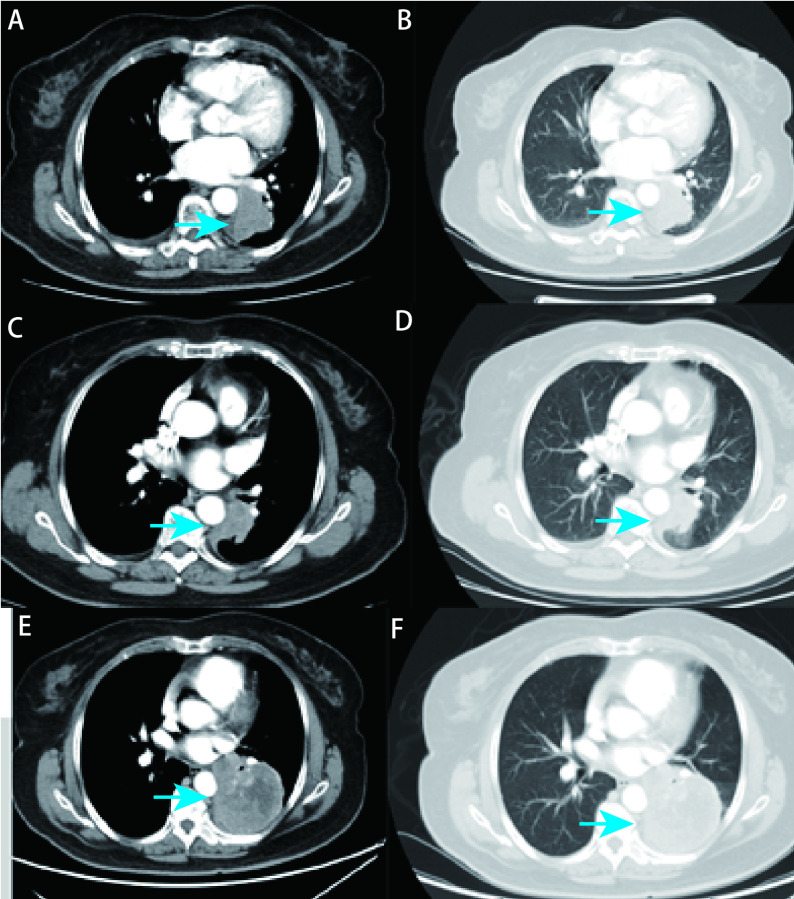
病例1在诊断和治疗中不同时间节点的胸部CT表现。A、B：患者确诊时的胸部CT表现，左肺下叶占位，病灶大小为46 mm×37 mm（A：纵隔窗；B：肺窗）；B：患者确诊时的胸部CT表现，左肺下叶占位，病灶大小为46 mm×37 mm；C、D：靶向治疗9个月后，病灶较前缩小，为39 mm×31 mm（C：纵隔窗；D：肺窗）；E、F：确诊为小细胞肺癌，病灶较前明显增大，77 mm×81 mm（E：纵隔窗；F：肺窗）。 Chest CT findings of case 1 at different time points during diagnosis and treatment. A, B: Chest CT findings of the patient at the time of diagnosis, mass in the lower lobe of the left lung, lesion size of 46 mm×37 mm (A: mediastinum window; B: lung window); C, D: After 9 months of targeted therapy, the lesion was 39 mm×31 mm smaller than before(C: mediastinum window; D: lung window); E: Diagnosed as lung small cell carcinoma, the lesion was significantly enlarged, 77 mm×81 mm (E: mediastinum window; F: lung window). CT: computed tomography.
